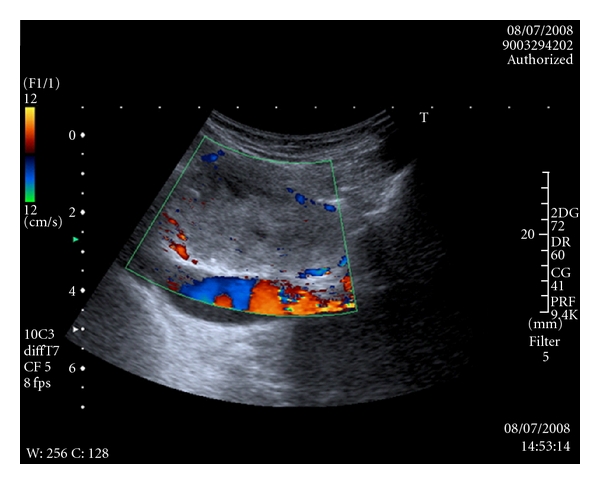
Colour flow Doppler ultrasound image of superior mediastinal mass.
